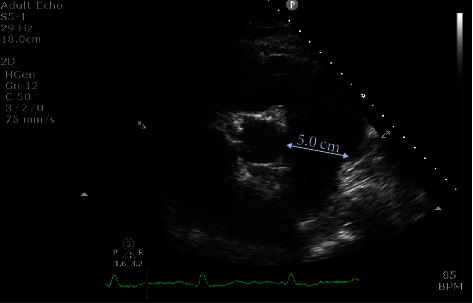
Transthoracic echocardiogram demonstrating significant dilatation of the main pulmonary artery (diameter: 5 cm).
